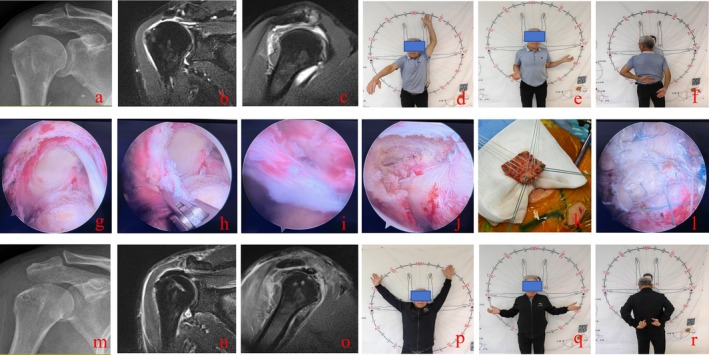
Patient, male, 57 years old, presenting with a massive irreparable rotator cuff tear. (a–c) Preoperative X‐ray and MRI showed a massive and irreparable rotator cuff injury, accompanied by upward displacement of the humeral head. (d–f) Preoperative shoulder joint range of motion during flexion, external rotation, and internal rotation. (g–j) Arthroscopy showed the conditions of the supraspinatus muscle, infraspinatus muscle, and subscapularis muscle. (k, l) Intraoperative preparation of the “sandwich” patch and rotator cuff repair. (m–o) The X‐ray and MRI results from the postoperative follow‐up examination showed that the rotator cuff repair was in good condition, the humeral head had shifted downward, and the transplanted tissue showed good signal. (p–r) Postoperative follow‐up shoulder joint range of motion for flexion, external rotation, and internal rotation.
